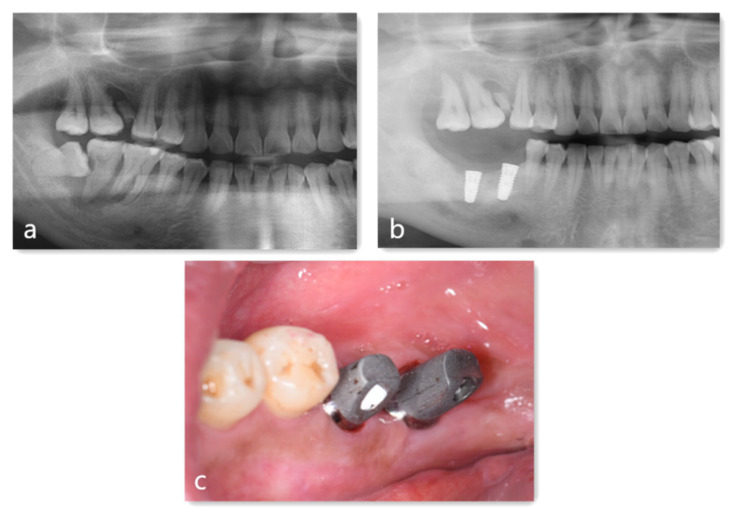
The treatment process of the case report. (a) Preoperative panoramic radiograph; (b) the panoramic radiograph 6 months after surgery; (c) Attaching abutments.
